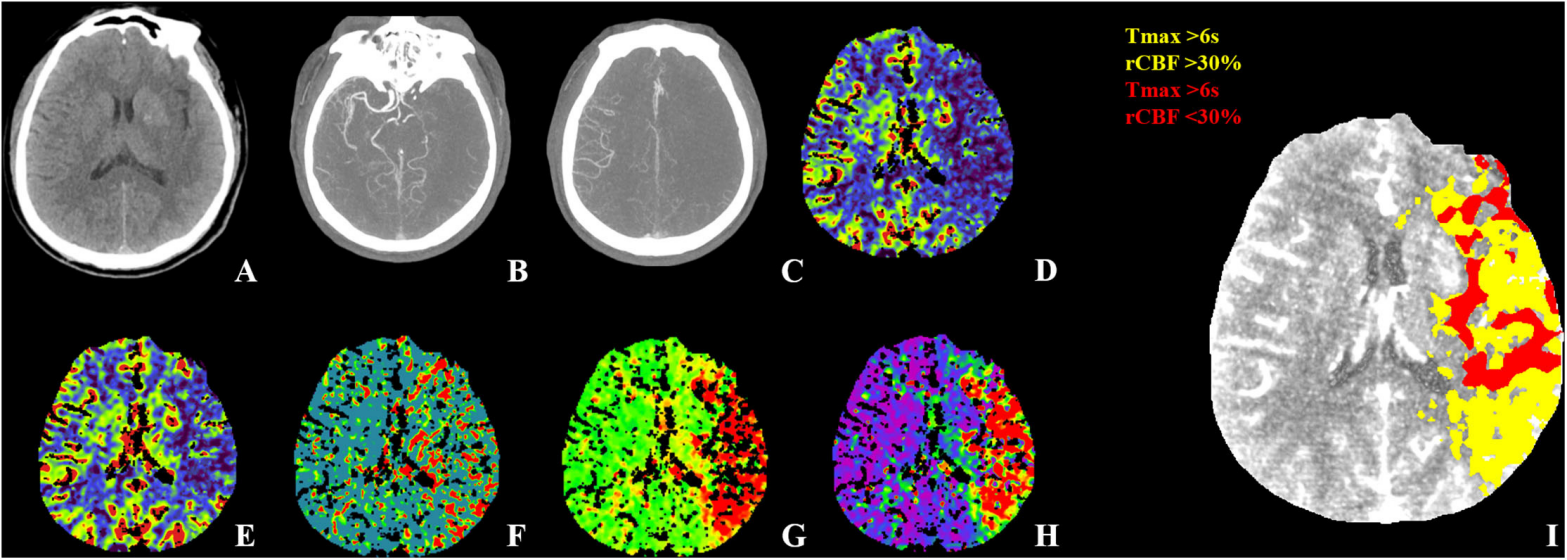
WUS with poor outcome. Fifty-seven-year-old male patient WUS patient last seen well 6.7 h before imaging. (A) The NECT show hypodensity in left the insular, lenticular nucleus, M1 and M2 with ASPECTS 6. (B) The CTA-MIP showed left ICA, M1 and M2 occlusion with CBS 0. (C) CTA-MIP showed collateral filling nearly 0% of the MCA flow territory with collateral score one. The CTP showed (D) a large area with decreased CBF, (E) CBV and (F–H) a large area with increased MTT, TTP, and Tmax. The CTP summary map (I) showed 93.73 ml of Tmax >6 s (yellow) and 59.08 ml of <30% rCBF decrease (red). The patient was treated EVT (mTICI 2b) and showed poor recovery with a 90-day mRS of six. WUS, wake-up stroke; ASPECTS, Alberta Stroke Program Early CT Score; MIP, maximum intensity projection; CBS, clot burden score; MCA, middle cerebral artery; EVT, endovascular thrombectomy; mTICI, modified Thrombolysis In Cerebral Infarction; mRS, modified Rankin Scale.
